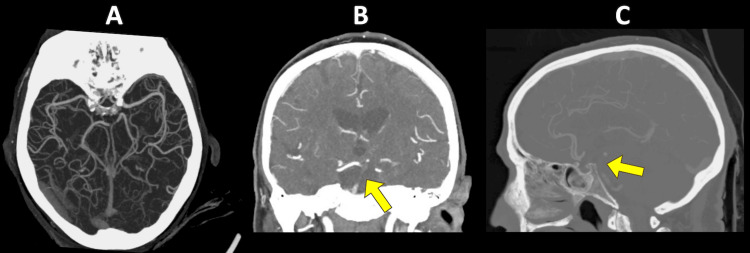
Occlusion not correctly identified as a large vessel occlusion by Viz LVO A 86-year-old female patient presented with basilar occlusion after a fall. Admission NIHSS score was 8. She was successfully revascularized with thrombectomy. The 24-hour post-intervention NIHSS score was 1. A) Viz LVO analysis on axial view of CT angiography helical reconstruction. B) Coronal view of non-contrast CT angiography with basilar occlusion (arrow). C) Sagittal view of non-contrast CT angiography with basilar occlusion (arrow). LVO, large vessel occlusion; NIHSS, National Institute of Health Stroke Scale; CT, computed tomography.
